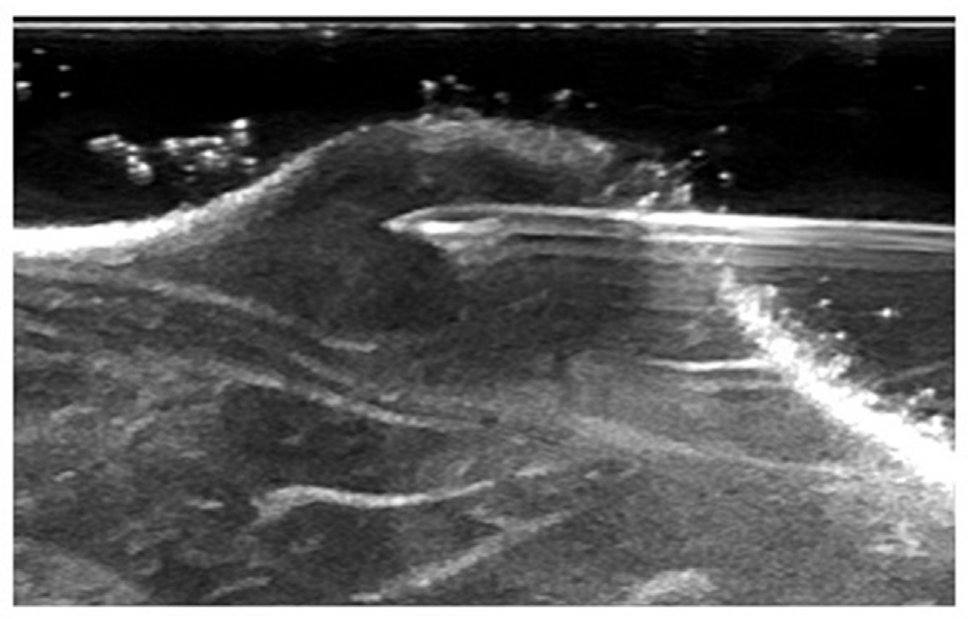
The needle was inserted horizontally into the center of the tumor under ultrasound guidance.
